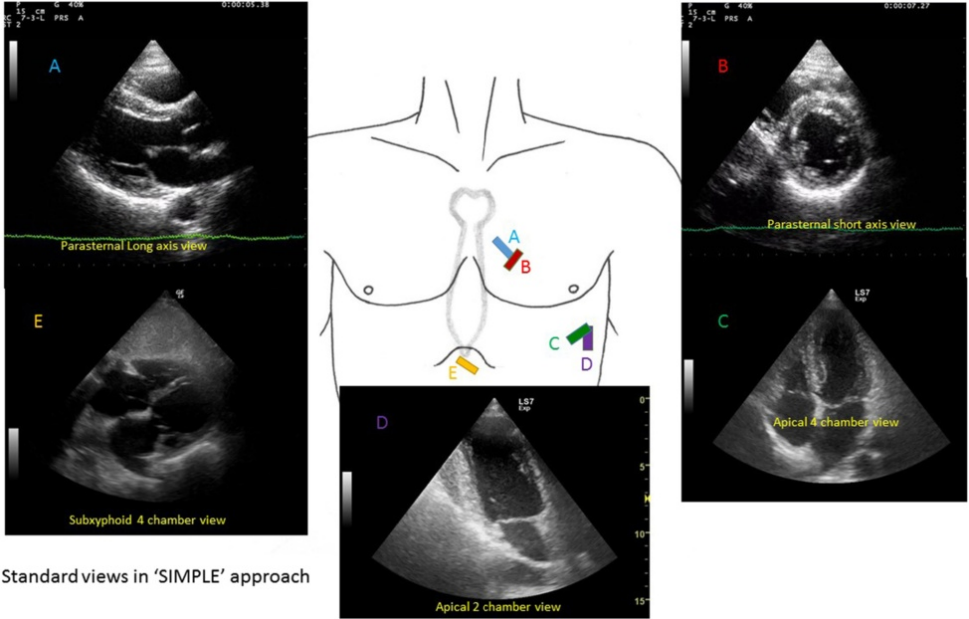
Five standard views used in focus echocardiography. They include parasternal long axis (a), parasternal short axis (b), apical four-chamber (c), apical two-chamber (d), and subxyphoid four-chamber views (e)
